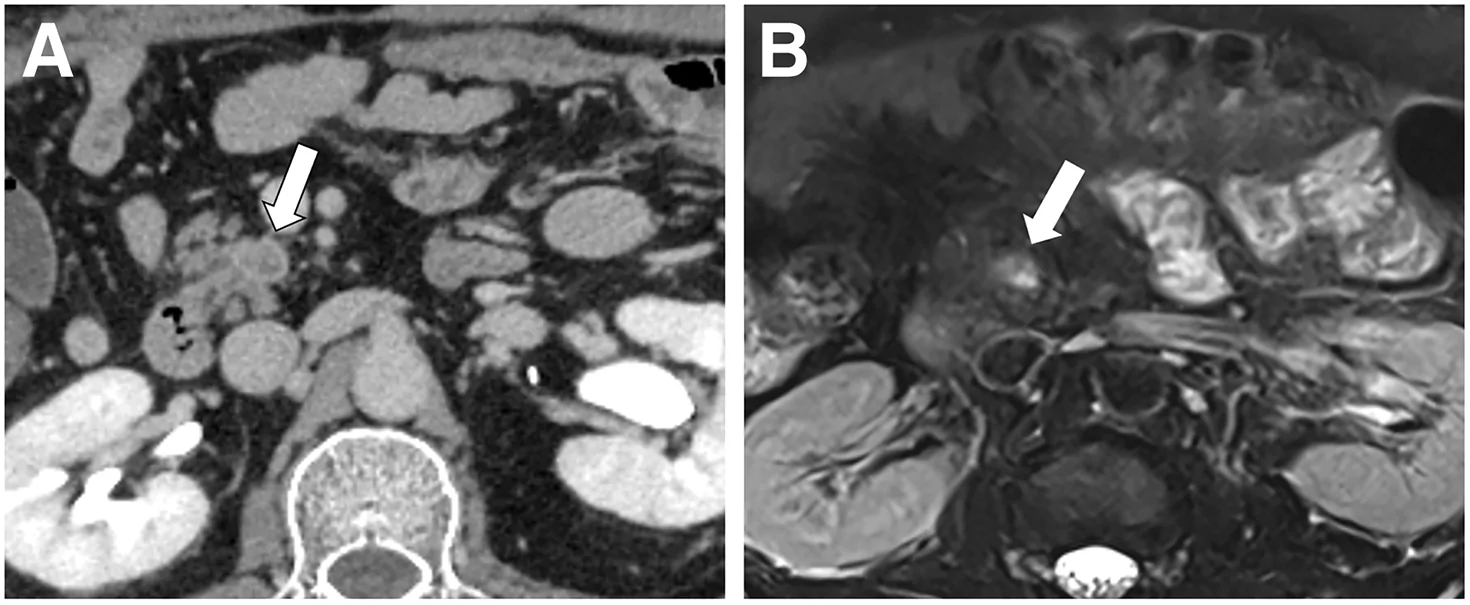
Imaging findings after NAC. (A) A newly identified hypoattenuating lesion in the pancreatic head on CT. (B) Diffusion restriction at the corresponding site on MRI. NAC, neoadjuvant chemotherapy
